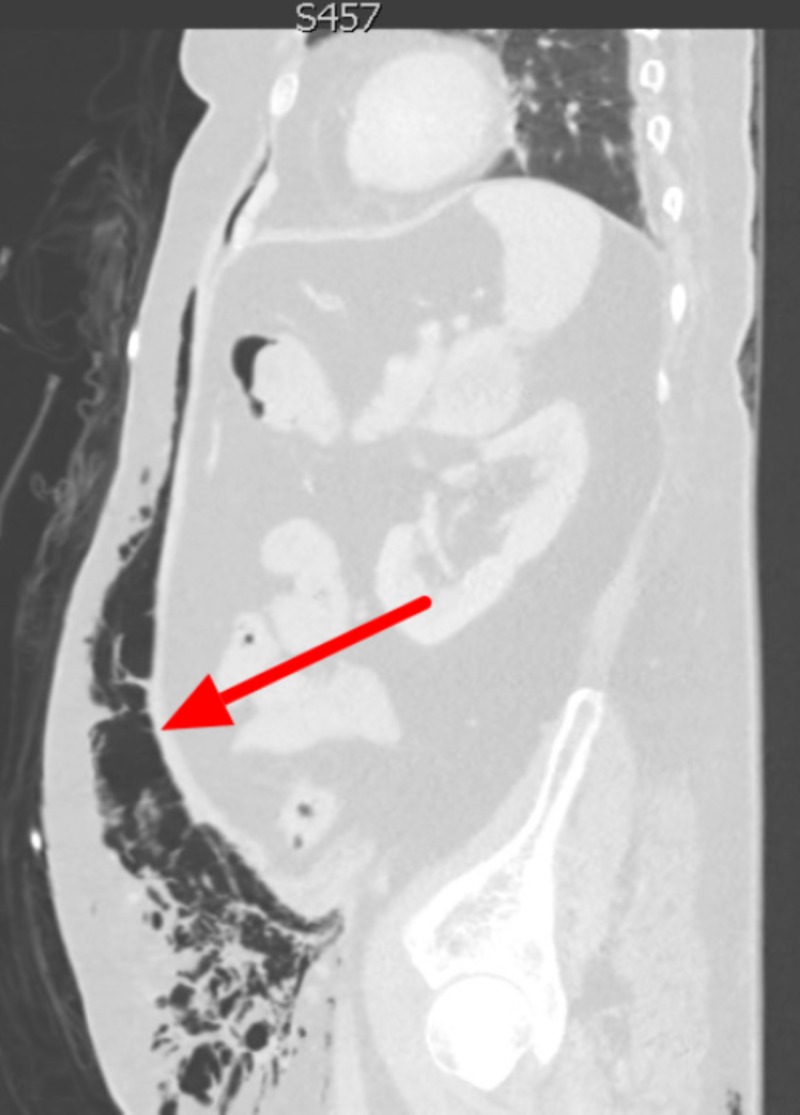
Sagittal CT image showing extensive soft tissue gas tracking along the patient’s entire left abdominal wall (red arrow) down to the thigh.
